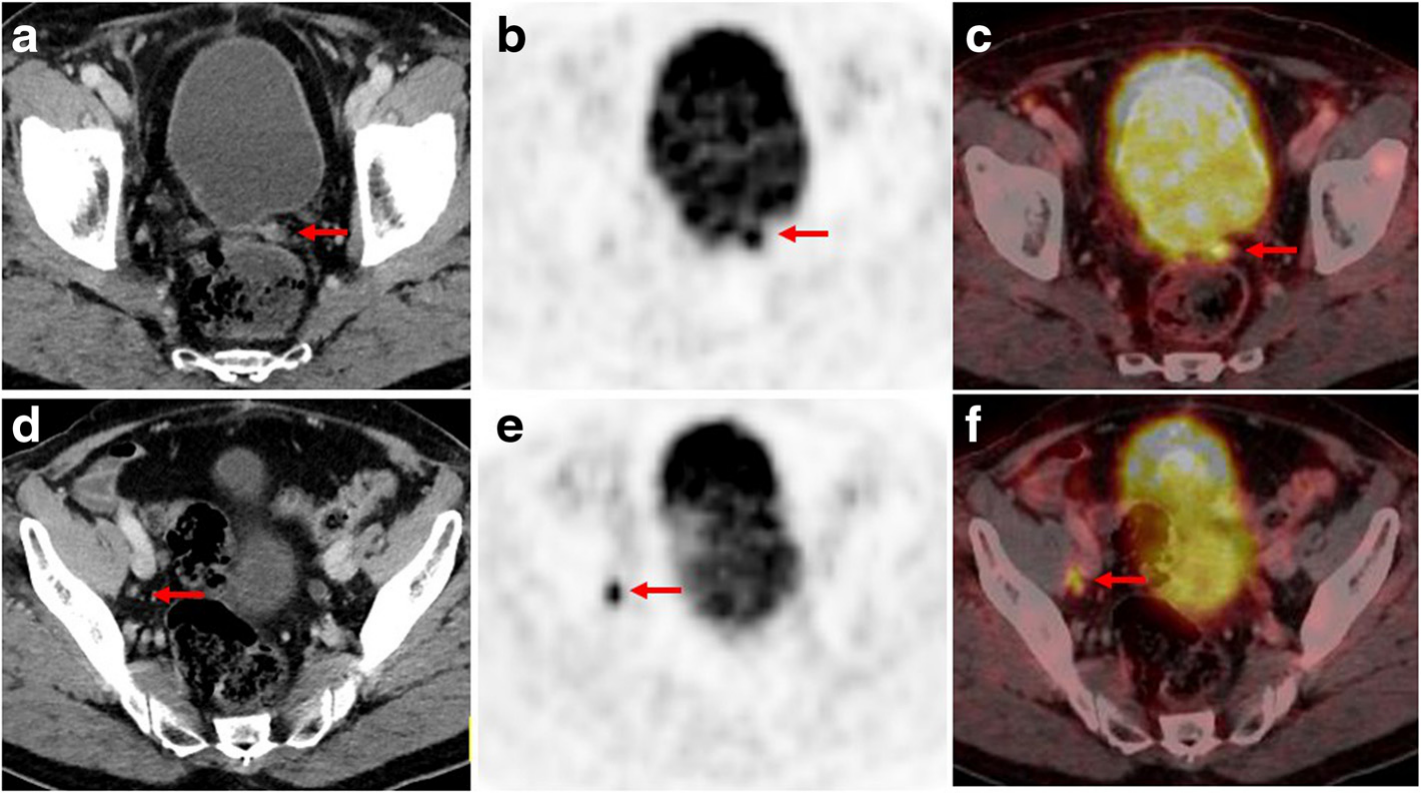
^68^Ga-PSMA ligand PET/CT examination of a 76-year old patient after radical prostatectomy (Gleason score 8, pT2b, pN1) followed by subsequent external beam radiation. 5 years later the patient presented with a rising PSA-value of 0.54 ng/ml. CT images show a 10 × 8 mm soft tissue formation in the former left prostatic bed a and a morphologically unsuspicious 4 mm lymph node (short axis) d dorsal of the right external iliac vein. Corresponding PET and fused PET/CT images show an intense, focal PSMA-ligand uptake in the former left prostatic bed b, c indicating a local recurrence as well as an intense, focal PSMA-ligand uptake in the small-sized lymph node dorsal of the right external iliac vein e, f indicating a lymph node metastasis
